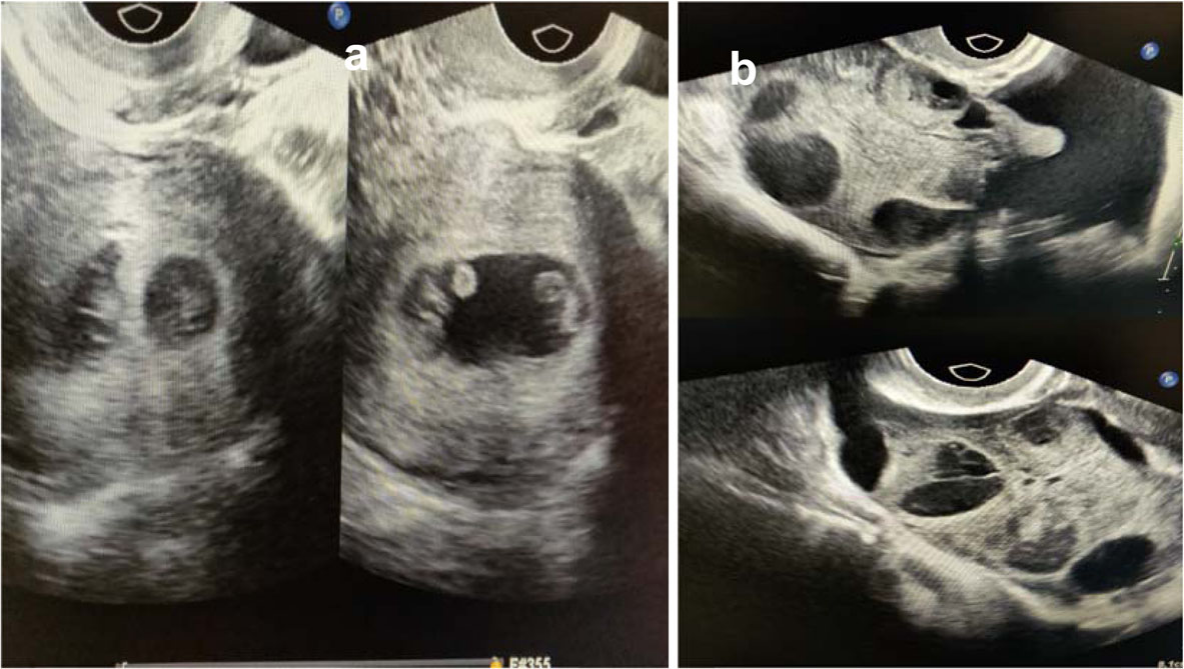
Two chorionic sacs were visualized by transvaginal ultrasound without any inter-twin membrane between two of the triplets. b Transvaginal ultrasonography of bilateral enlarged multicystic ovaries (Upper: Left ovary Lower: Right ovary)
